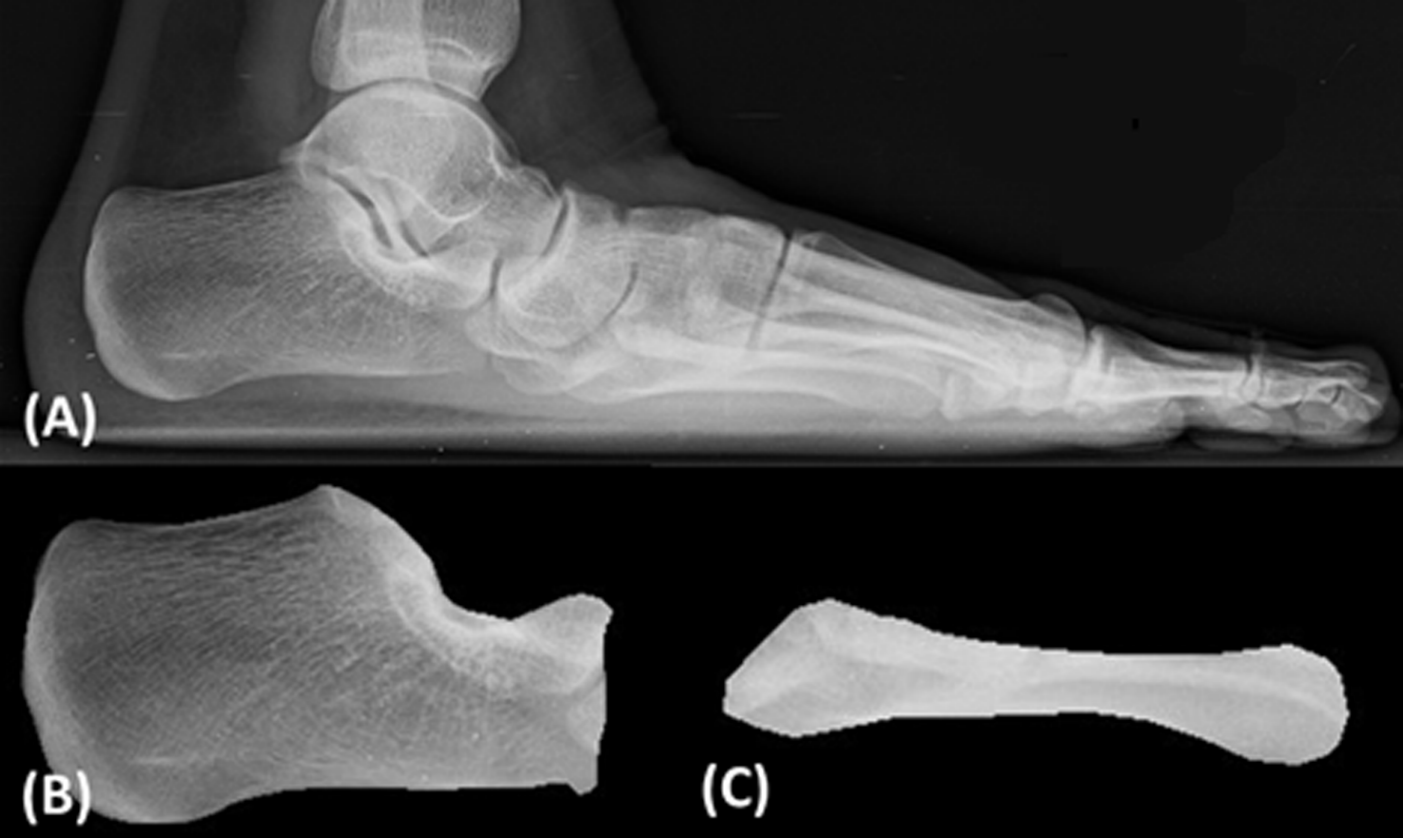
Lateral radiographic image of a participant. (A) The original radiographic image. The reference images of the calcaneus (B) and the fifth metatarsal bone (C) isolated from (A).
